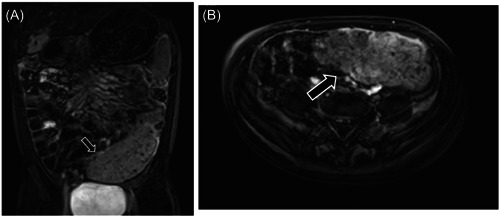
(A) MRI enterography image revealed a hyper‐vascular mass in the area of the sigmoid colon. (B) Transverse view of the sigmoid colon mass.
